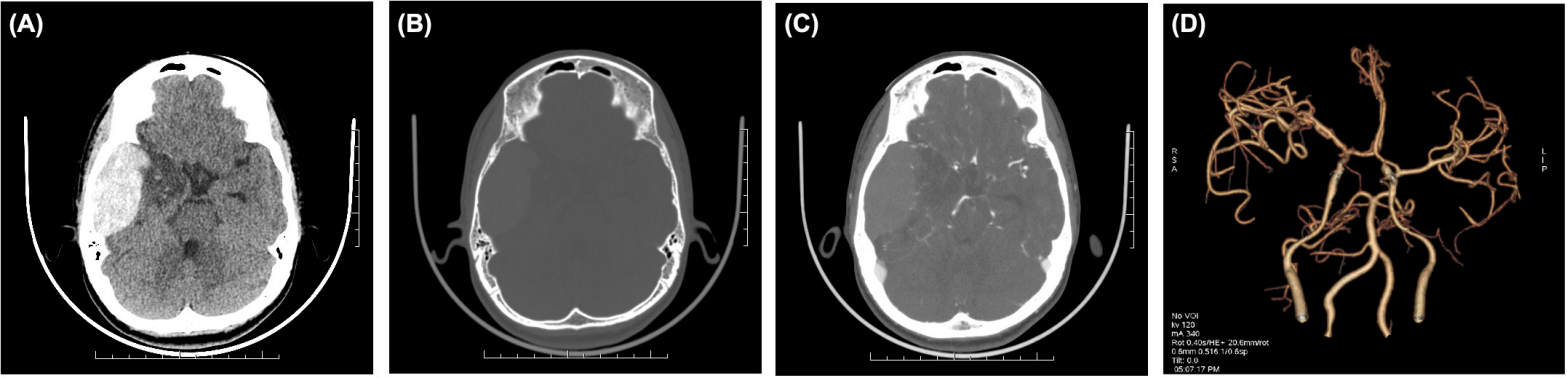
(A) Non‐contrast computed tomography (NCCT) scan axial section of head, brain window demonstrates hyperdense blood attenuation extradural collection in the right temporal region with mass effect and effacement of ipsilateral lateral ventricle and sulcal spaces. (B) Non‐contrast computed tomography (NCCT) scan axial section of head, bone windows do not show any fracture or bone pathologies. (C) Contrast enhanced computed tomography (CECT) arterial phase demonstrate displacement of cranial vessels due to extradural collection. No any vascular abnormalities are noted. (D) Reconstructed Volume rendering technique (VRT) shows no any abnormality.
